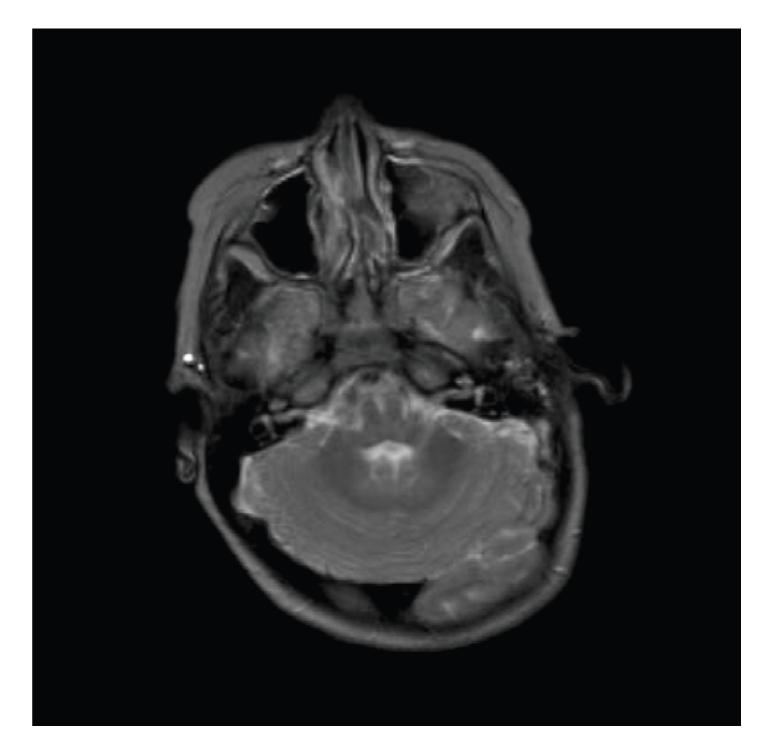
Magnetic resonance axial T2-weighted imaging in a patient with rhombencephalosynapsis shows fusion of the vermis and cerebellar hemispheres.
